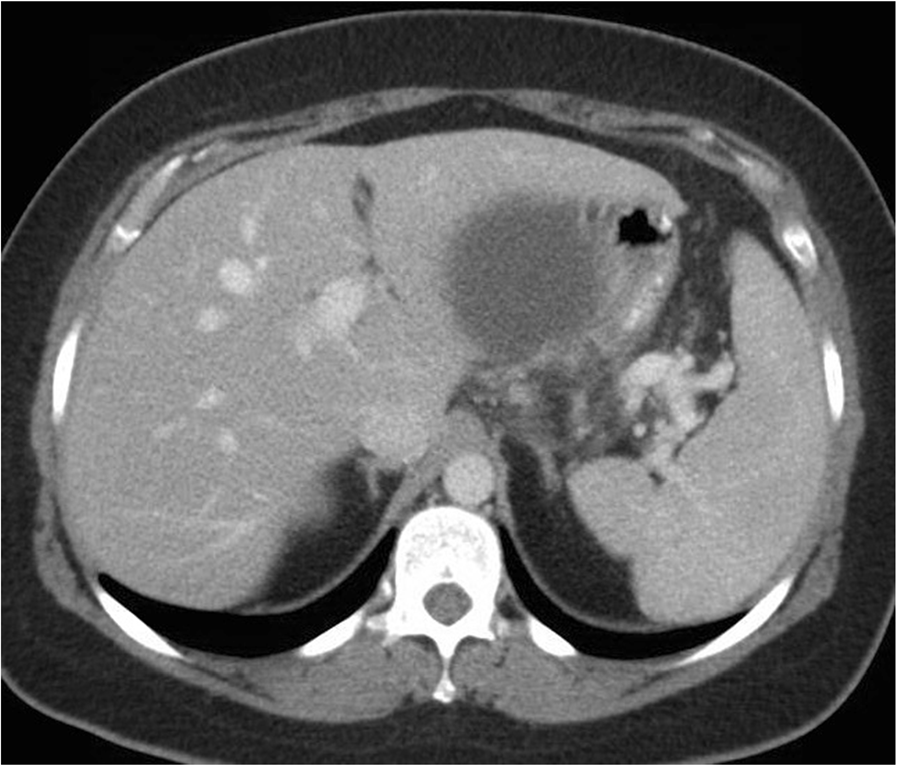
Sleeve compression (axial CT image)
